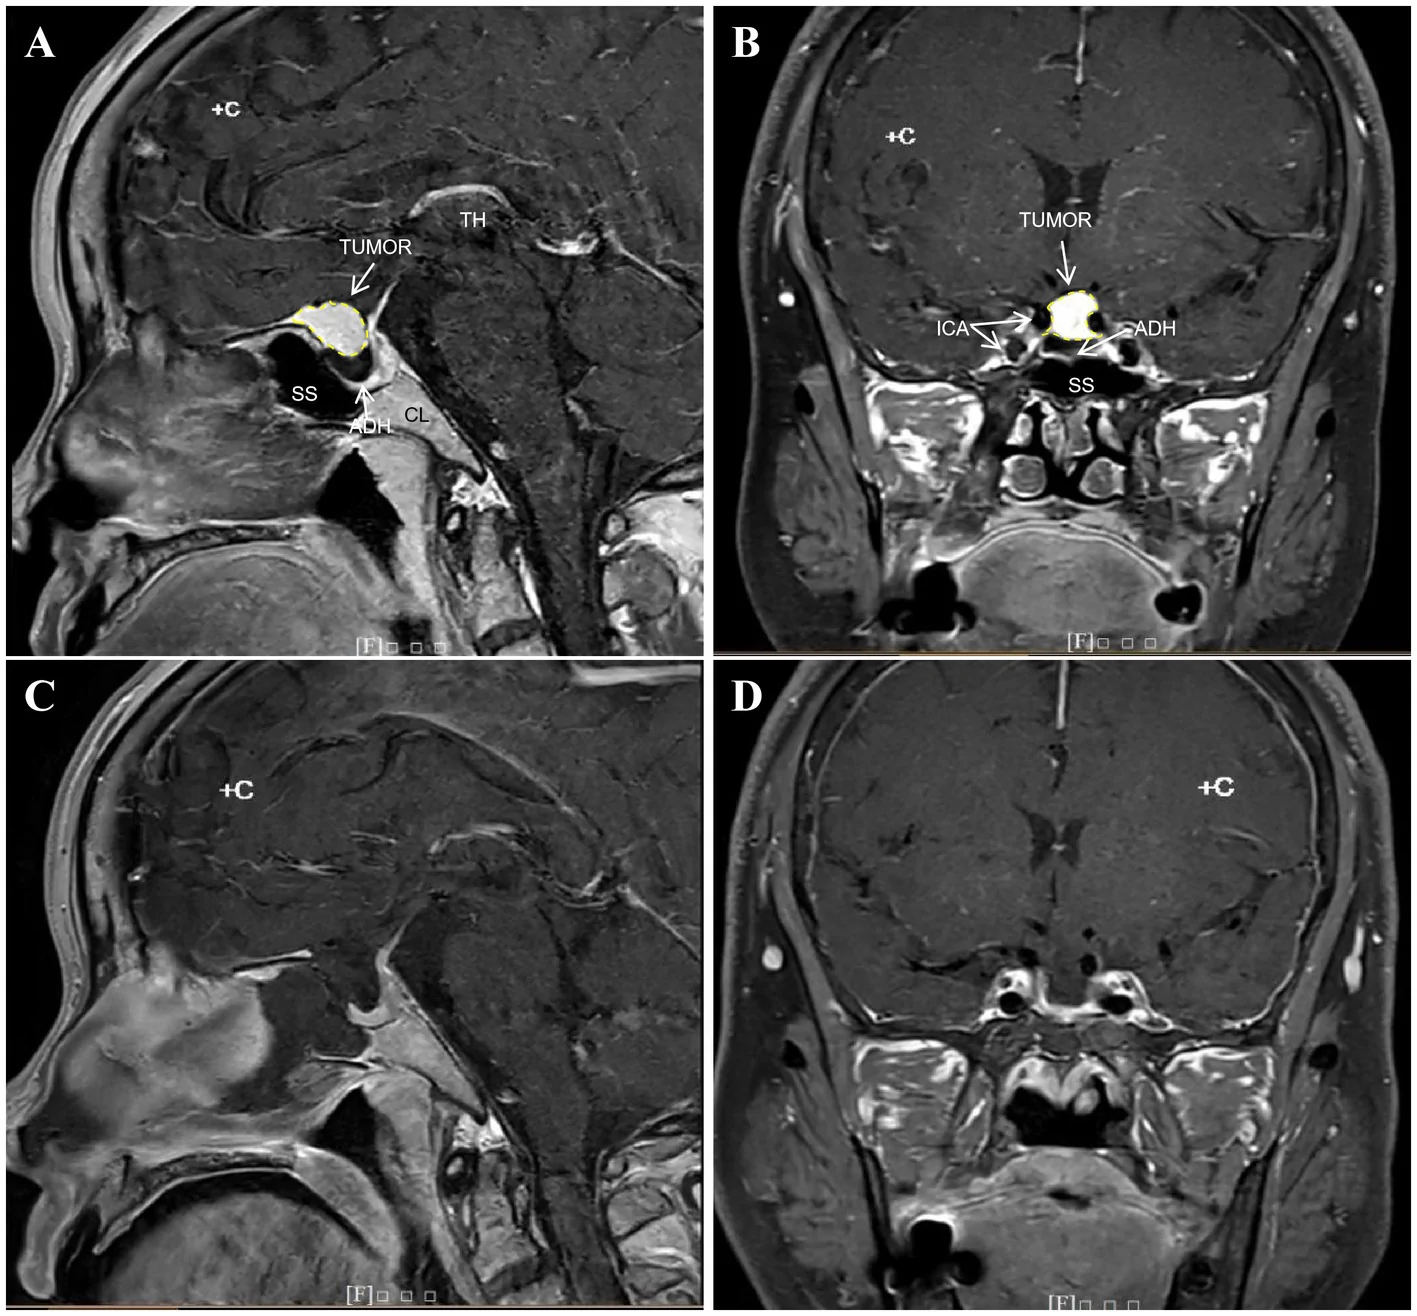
Preoperative and postoperative MRI of Patient 2. (A,B) Preoperative sagittal and coronal images demonstrating a Tuberculum Sellae meningioma. (C,D) Postoperative images showing complete tumor removal. TH, thalamus; ADH, adenohypophysis; SS, sphenoid sinus; CL, clivus; ICA, internal carotid artery.
